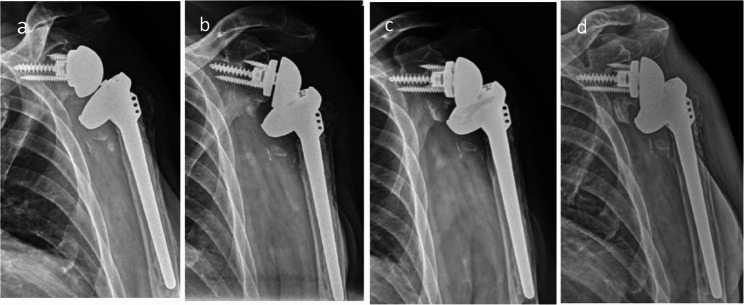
Case 2. a, b, c, d X-ray after 3 months, 6 months, 1 year, 1.5 years respectively
